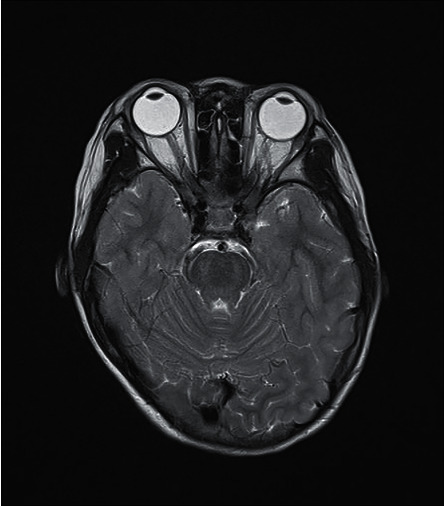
MRI brain showing T2 hyperintensities at posterior and bilateral pons.
